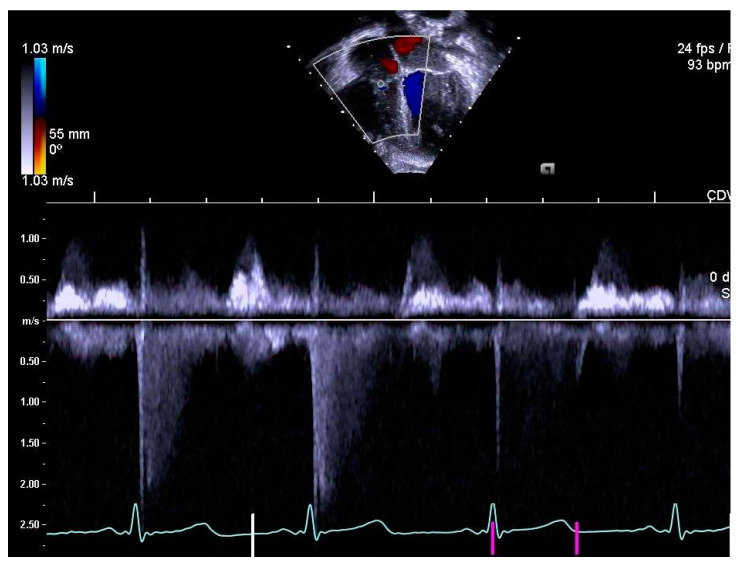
Tricuspid regurgitation (TR) measured by continuous wave Doppler.
